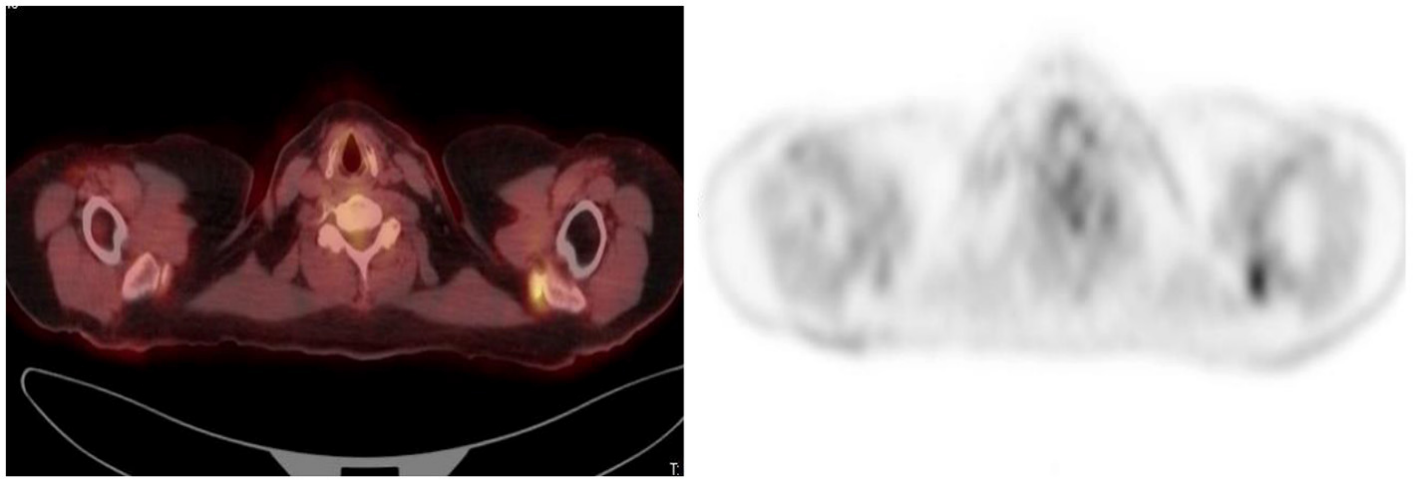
56 year old woman with ovarian Ca. Combined PET/CT and PET images show intense uptake in the left acromio-clavicular joint, which is osteodegenerative.
